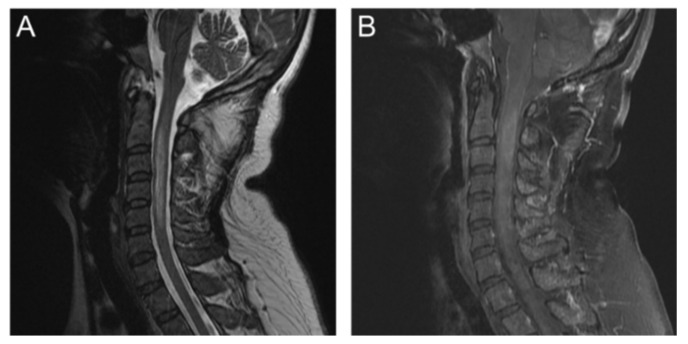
Initial conventional magnetic-resonance imaging MRI. (A) T2-weighted image (T2WI) showed mild spinal-cord swelling with hyperintensity lesion in C2–C5 spinal cord; (B) postcontrast T1-weighted image (T1WI) with fat suppression showed intramedullary lesion with faint enhancement in C2–C3 spinal cord.
